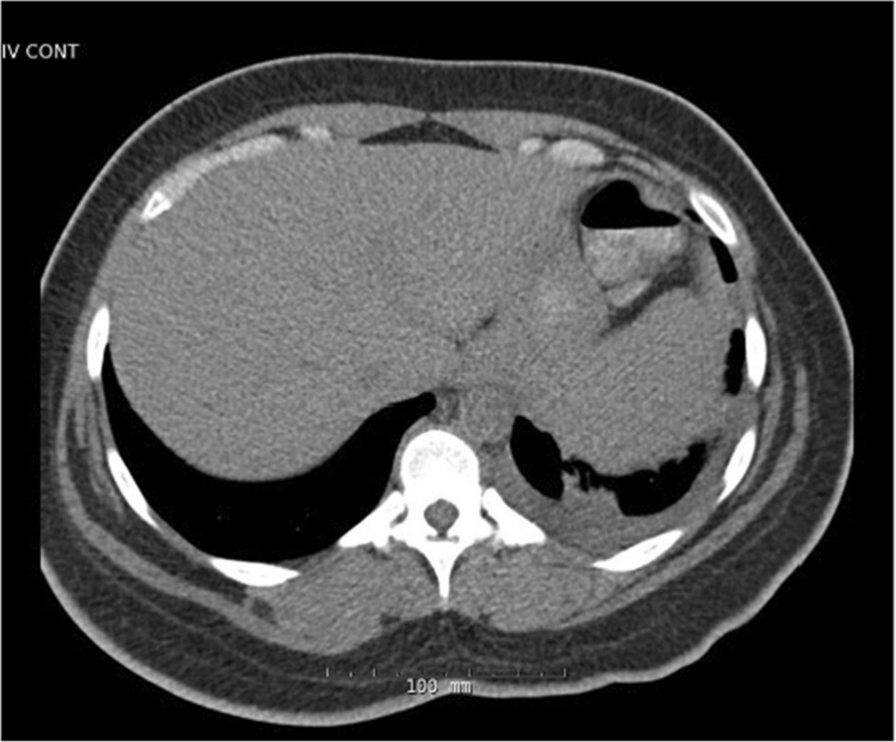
Computed tomography chest, abdomen, pelvis with small pleural effusion and atelectasis at left lung base
